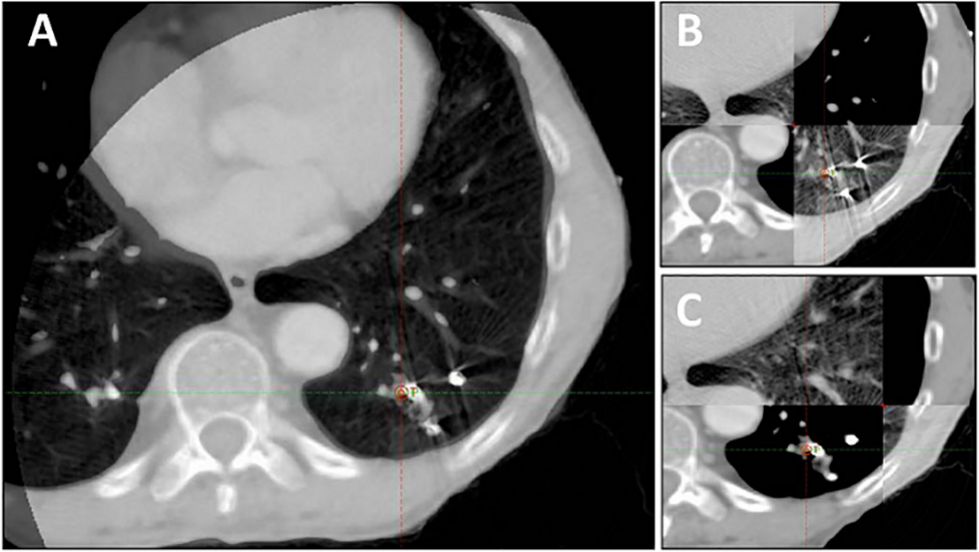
Breath-hold CT and CBCT (spotlight) matching with three fiducial markers in the left lower lobe of the lung, just prior to SBRT treatment. Panel A shows CBCT completely overlaid onto the planning CT images. Panel B-C shows the two-way sliding window matching between CBCT and planning CT images.
